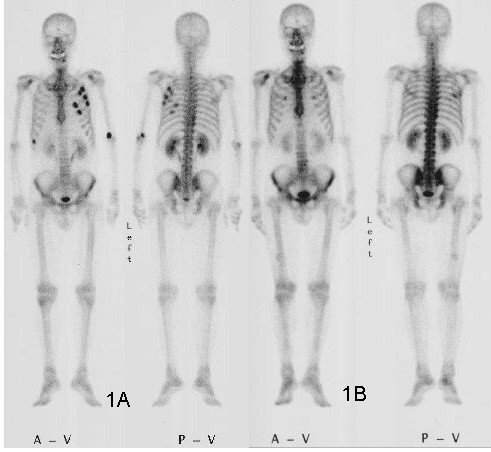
A) 99mTc-HMDP bone scintigraphy showing many lesions with accumulation in the left ribs, which were diagnosed as multiple costal metastases. B) After chemotherapy with S-1 and CPT-11, the costal metastases have resolved.
